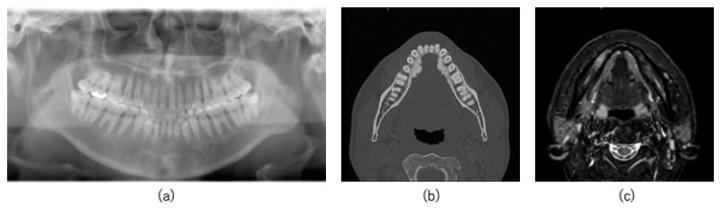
Preoperative imaging findings. (a) Preoperative panoramic radiograph. No significant bone destruction is observed. (b) Infiltration is observed in the cortical bone in the mandibular right second premolar region. (c) Short tau inversion recovery magnetic resonance imaging (MRI) displaying a high signal intensity area measuring 23 mm anteroposteriorly in the mandibular right first premolar to second molar region.
